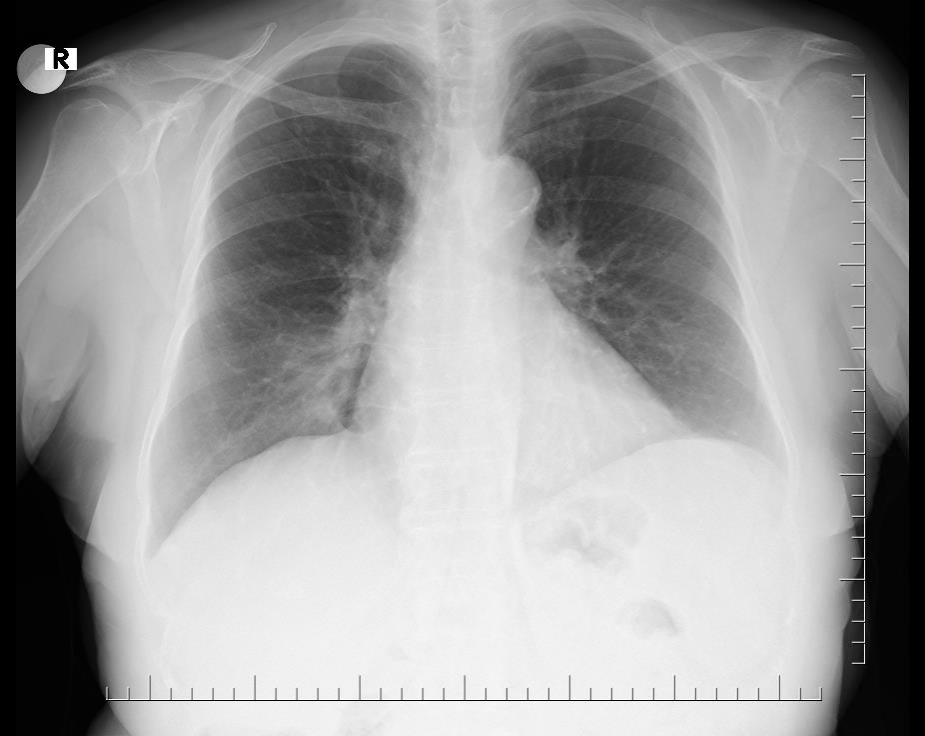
Chest X-ray showing right paracardiac ill-defined opacity.
